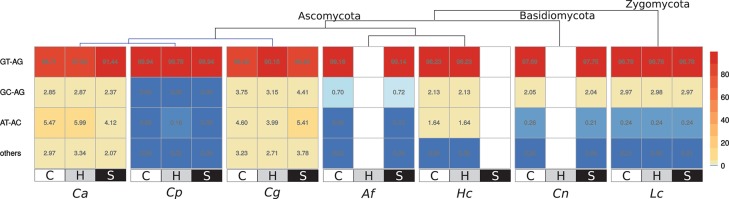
Used splice sites in the studied species (in %) during control (C), response-to-host (H) and stress (S) conditions for each species are shown. “Others” summarizes the ratio of the splice sites CT-AC, CT-GC, GN-AG, and GT-AT.
